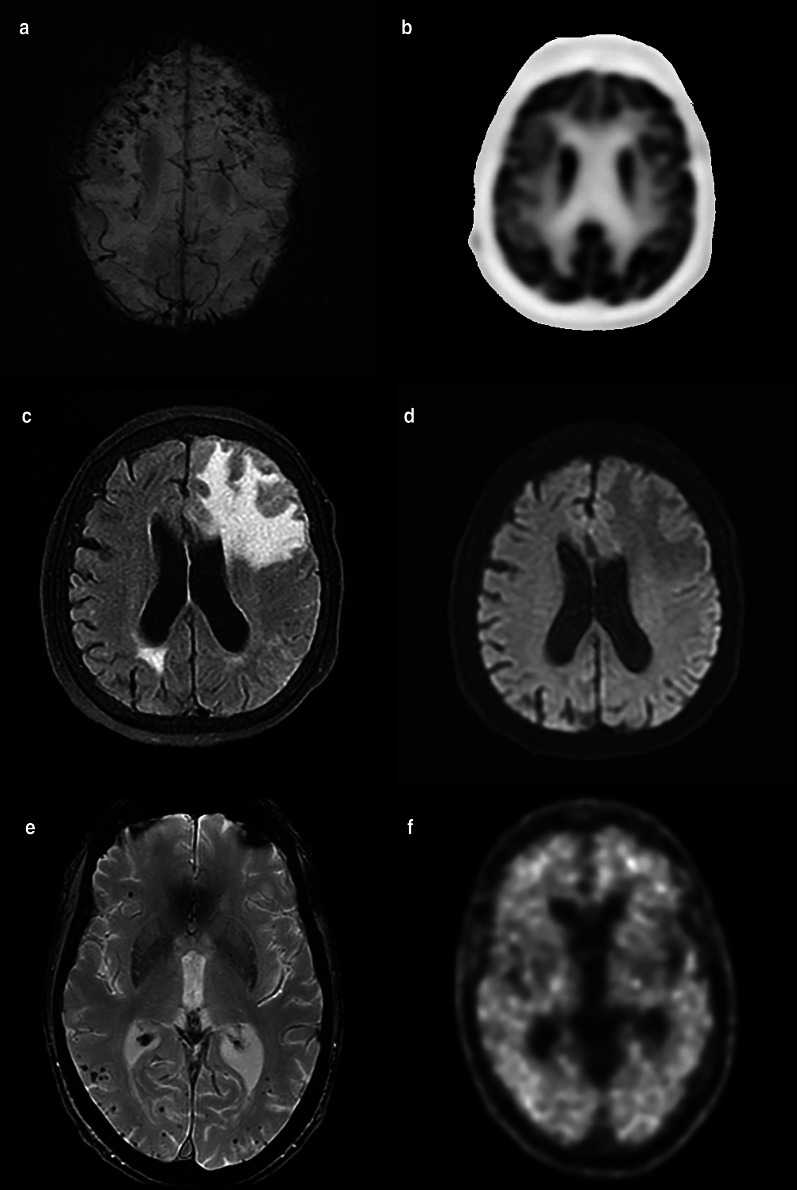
A 81-year-old female with a gradual decline in short term memory and depression. Susceptibility-weighted image demonstrates numerous subcortical microhemorrhages (a). Fluorodeoxyglucose PET/MRI (b) demonstrates relatively normal cerebral metabolism. Later she presented with an acute worsening of symptoms including new right-side weakness and dysphasia. Magnetic resonance imaging demonstrated T2 FLAIR hyperintensity within the left frontal lobe (c) without restricted diffusion (d). Final diagnosis was amyloid beta-related angiitis. Cognitive function improved following administration of steroids. Fluorodeoxyglucose PET/MRI suggested against concurrent AD. Images from a separate patient demonstrate occipito-temporal subcortical microhemorrhages (e) with diffuse amyloid uptake on florbetapir PET/CT, a pattern suggesting concurrent CAA with AD (f)
